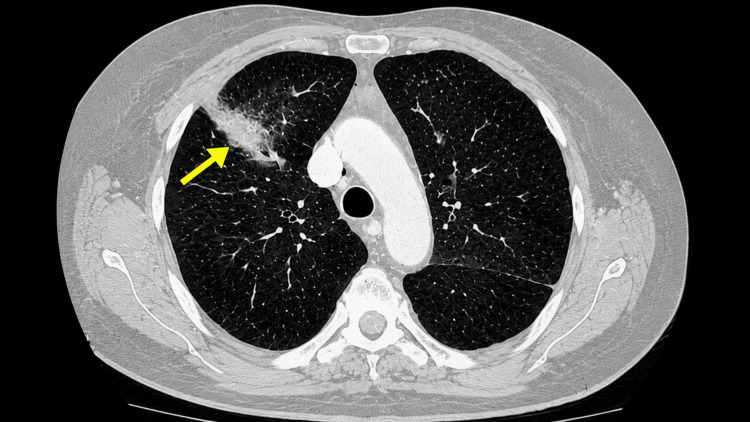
Axial CT pulmonary angiography (lung window) demonstrating a focal peripheral consolidation in the right upper lobe (arrow), which initially raised the differential diagnosis of pneumonia versus pulmonary infarction. CT: computed tomography
